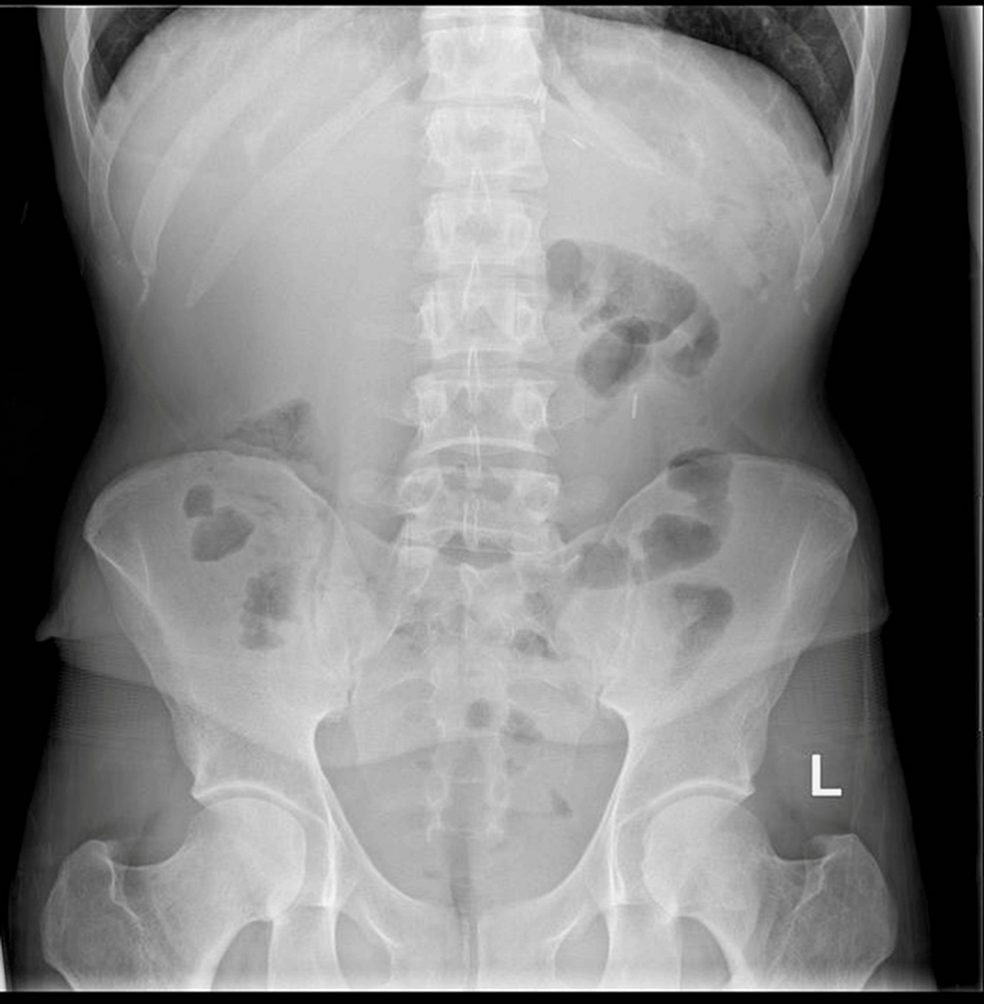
Abdominal X-ray showing normal findings.
